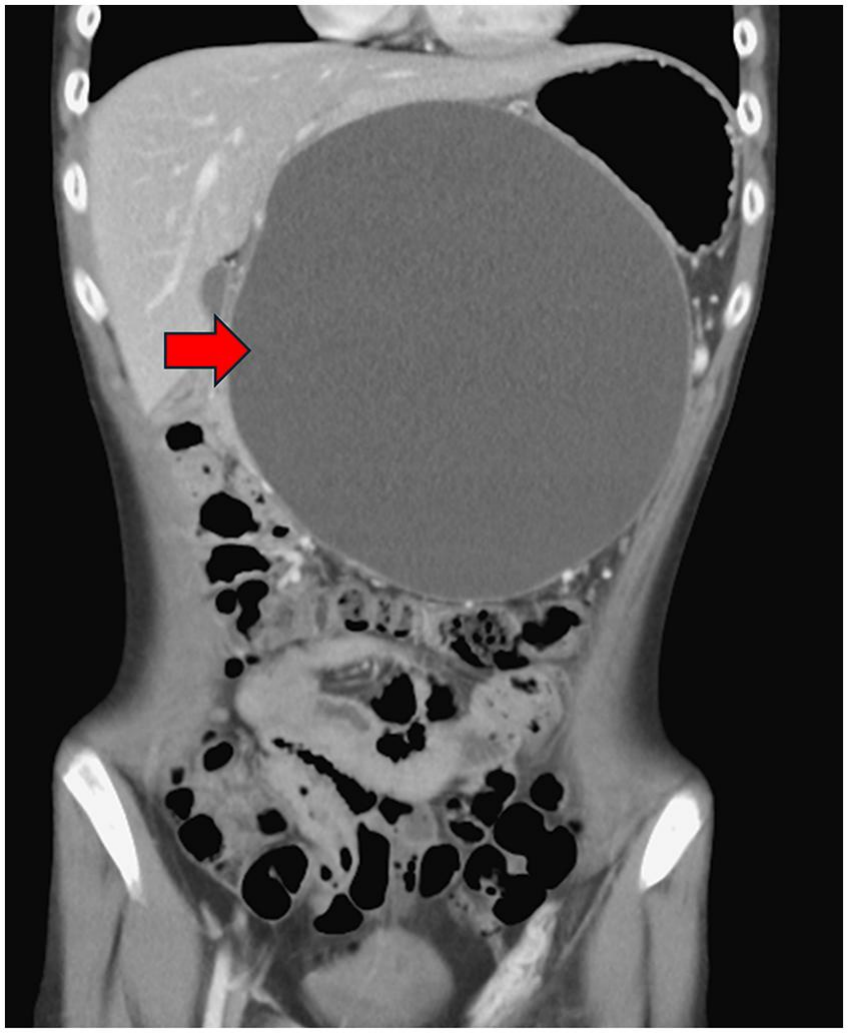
Abdominal computed tomography scan demonstrated pancreatic pseudocyst in coronal view at first visit at our hospital (red arrow).
